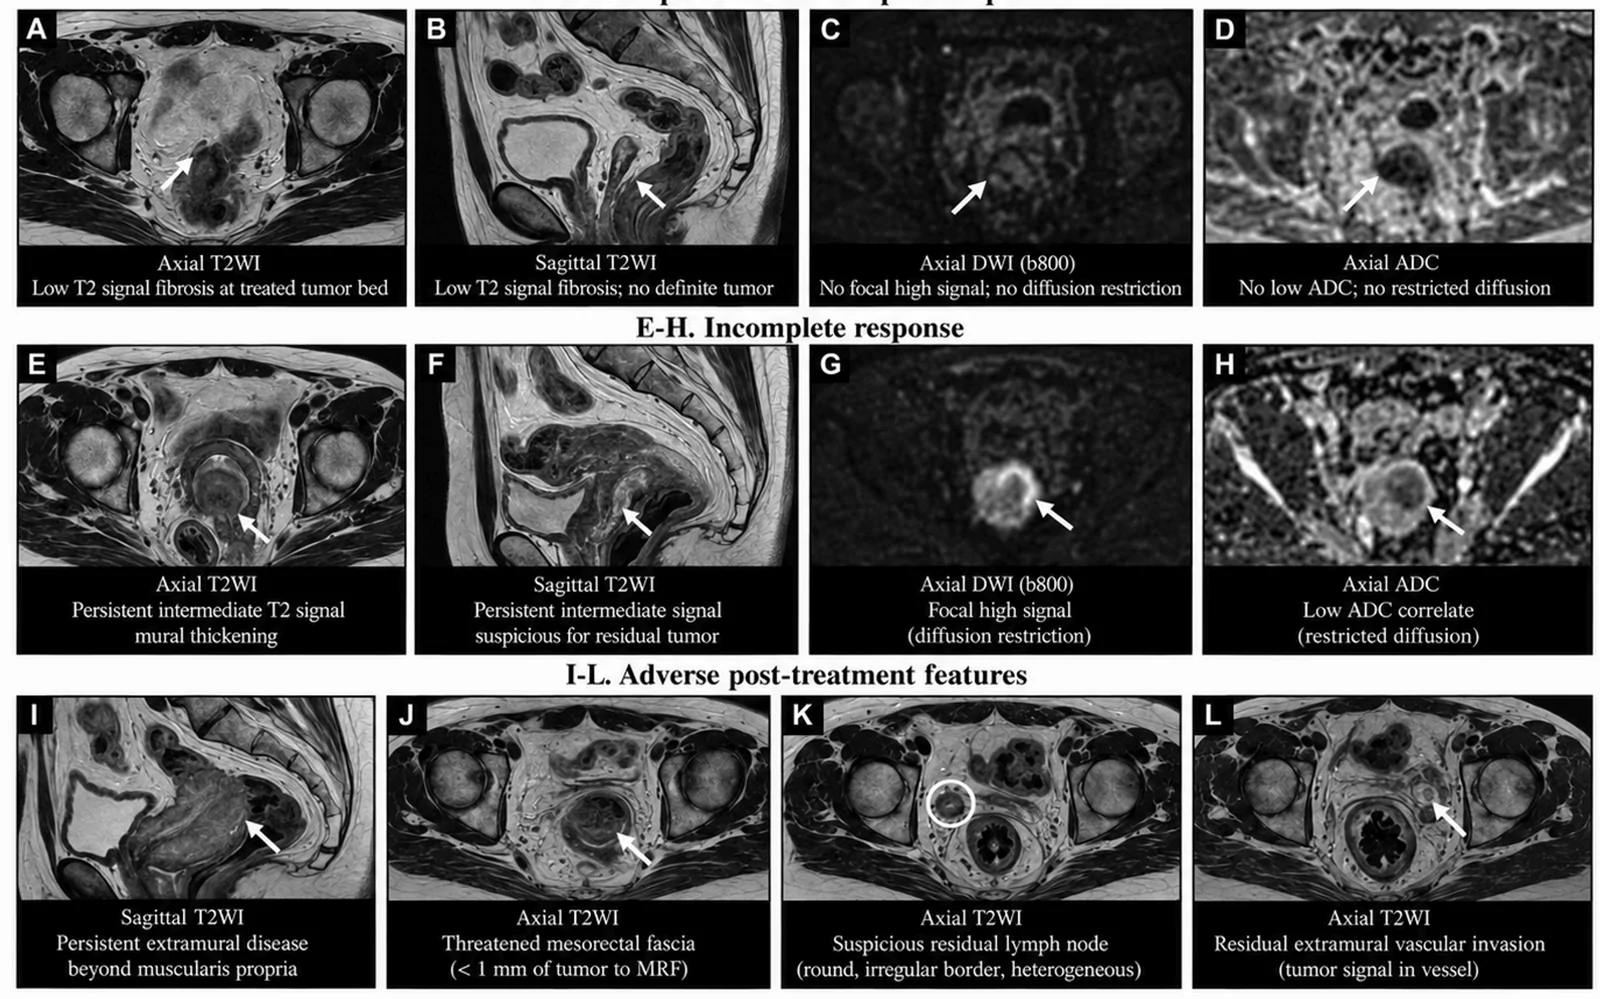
Representative post-treatment magnetic resonance imaging response patterns in rectal cancer. (A-D) Complete or near-complete response showing low T2 signal fibrosis at the treated tumor bed without definite focal diffusion restriction. Panel A shows axial T2-weighted imaging with low-signal fibrosis at the treated tumor bed. Panel B shows sagittal T2-weighted imaging with low T2 signal fibrosis and no definite residual tumor. Panel C shows axial diffusion-weighted imaging with no focal high signal. Panel D shows the corresponding axial apparent diffusion coefficient map without low signal, indicating absence of restricted diffusion. (E-H) Incomplete response showing persistent intermediate T2 signal and corresponding focal diffusion restriction suspicious for residual viable tumor. Panel E shows axial T2-weighted imaging with persistent intermediate T2 signal mural thickening. Panel F shows sagittal T2-weighted imaging with persistent intermediate signal suspicious for residual tumor. Panel G shows axial diffusion-weighted imaging with focal high signal at the treated tumor bed. Panel H shows the corresponding axial apparent diffusion coefficient map with low signal, indicating restricted diffusion. (I-L) Adverse post-treatment features. Panel I shows sagittal T2-weighted imaging with persistent extramural disease beyond the muscularis propria. Panel J shows axial T2-weighted imaging with threatened mesorectal fascia. Panel K shows axial T2-weighted imaging with a suspicious residual lymph node. Panel L shows axial T2-weighted imaging with residual extramural vascular invasion. ADC = apparent diffusion coefficient; DWI = diffusion-weighted imaging; EMVI = extramural vascular invasion; MRI = magnetic resonance imaging; T2WI = T2-weighted imaging Figure panels are representative imaging examples. No statistical comparison was performed for this figure.
